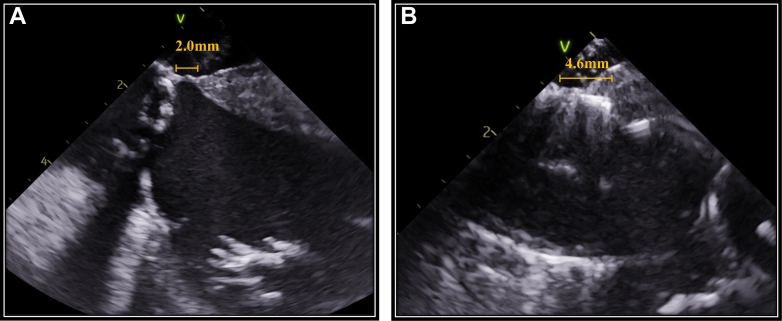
Intracardiac Echocardiography Findings in a Comparison Case With Valve Contact to the Muscular Septum (A) Preprocedural intracardiac echocardiography (ICE) image showing measurement of the membranous septum length. (B) ICE image after valve deployment showing that the prosthetic valve extended beyond the membranous septum and directly contacted the muscular septum.
